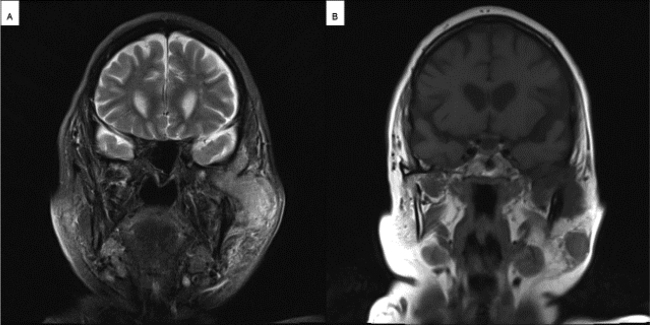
(A) Coronal T2 weighted MRI scan showing extensive temporomandibular growth of the left parotid tumor. (B) Coronal T1 weighted MRI scan showing the primary parotid tumor invading the temporomandibular joint and a synchronous tumor of the parotid tail.
